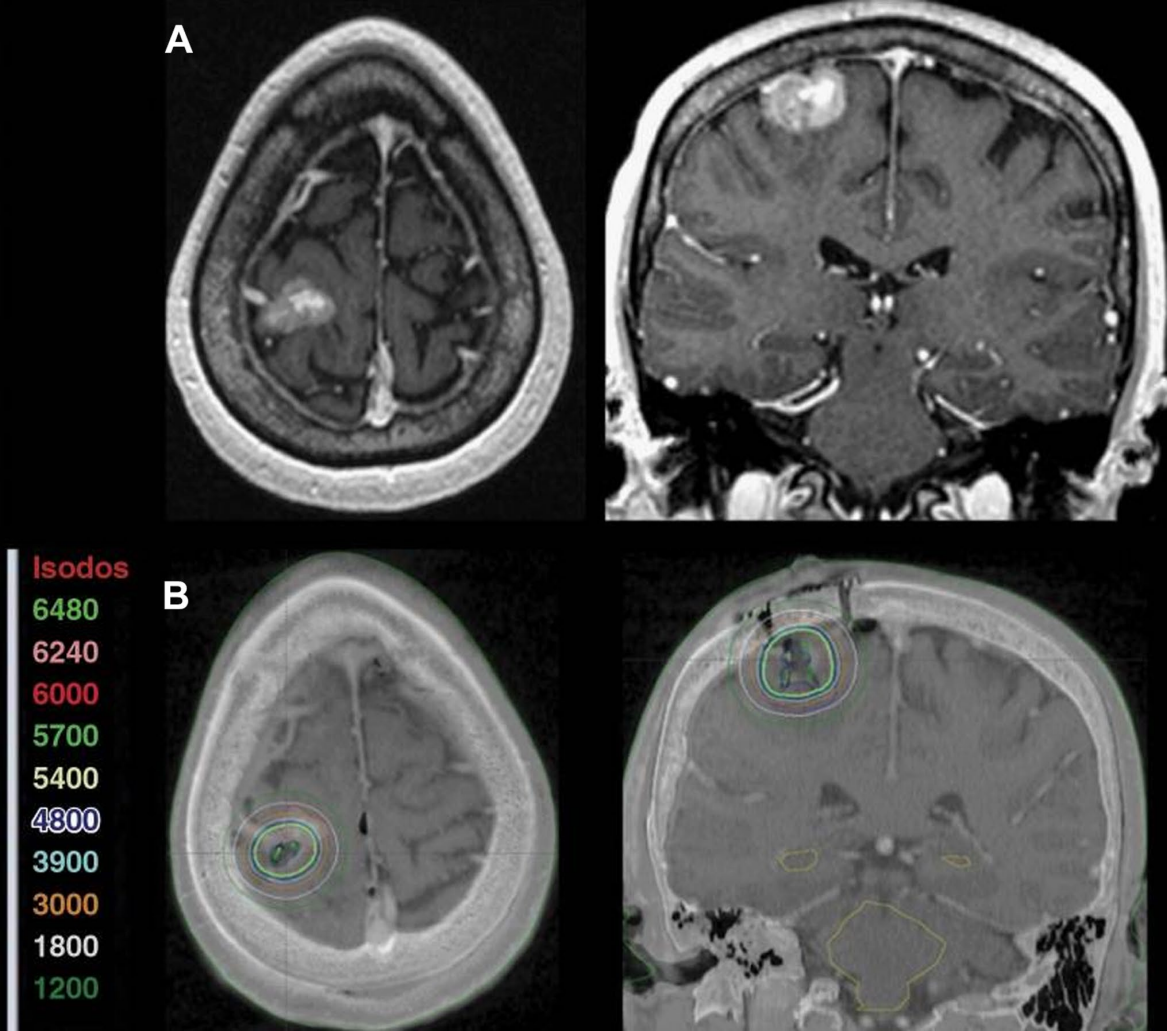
GammaTile dosimetry. A Preoperative T1 post contrast MRI showing a single brain metastasis. B Postoperative MRI fused with postoperative CT scan demonstrating GammaTile dosimetry (doses in centigray) [8]
